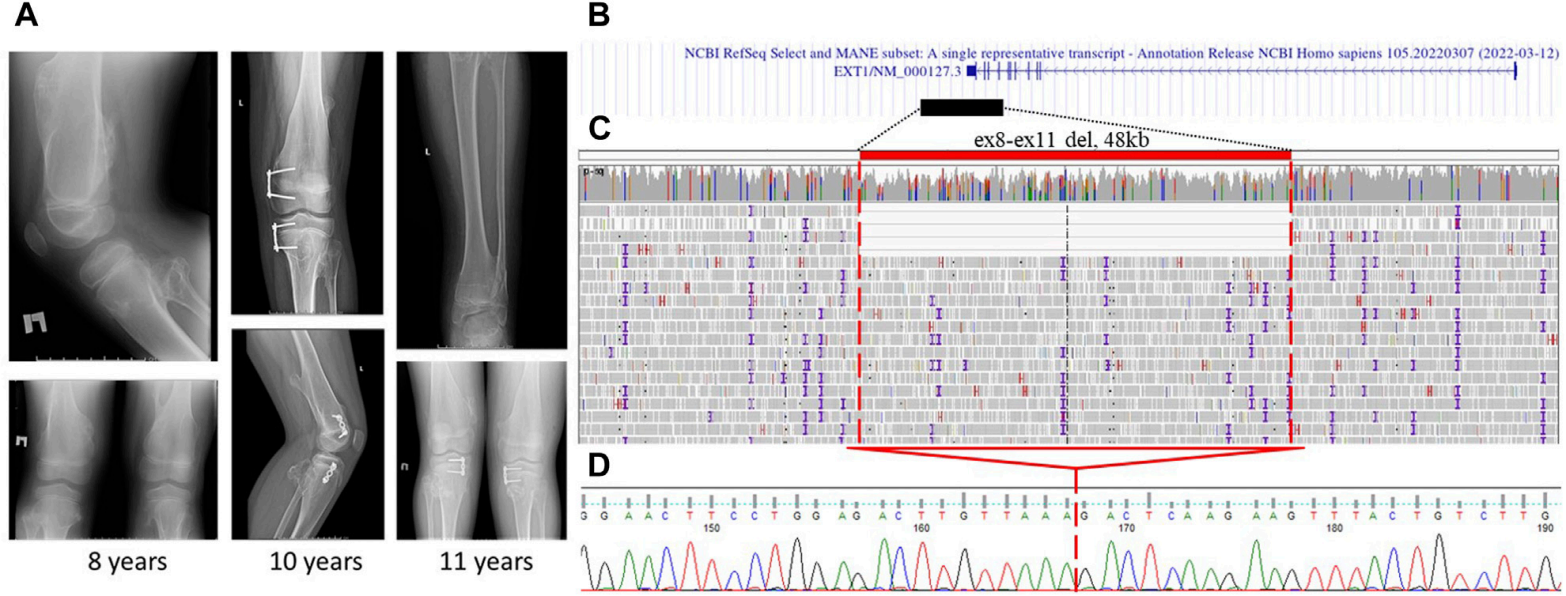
Case 1: (A) X-ray images of proband 1 at the age of 8, 10, and 11 years. Multiple osteochondromas around the knee and on the femur are shown. (B) Alignment of the deletion of exons 8–11 in the *EXT1* gene in the UCSC browser was found. (C) GS data on mosaic gross deletion from case 1 visualized in the IGV browser. (D) Chromatogram showing the region encompassing the breakpoints of the deletion, with a red line marking the borders of the deletion.
